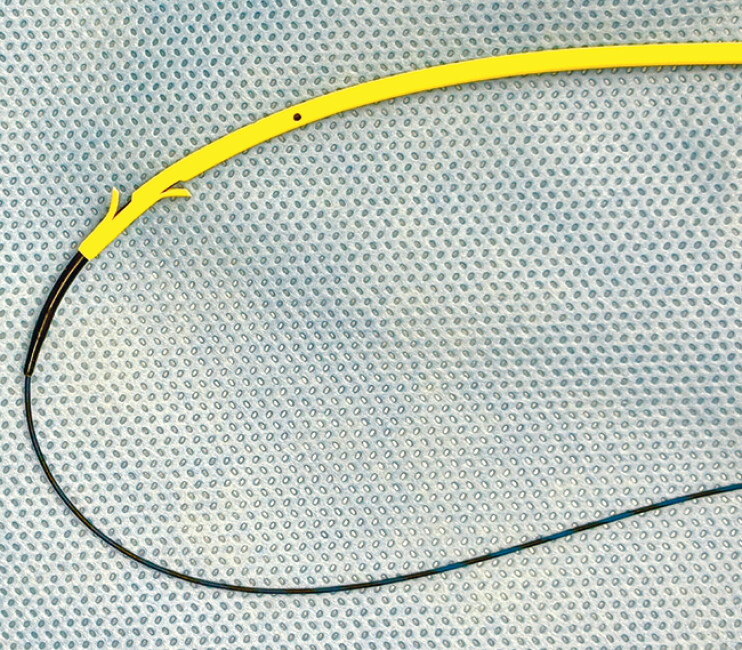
A novel 0.035-inch guidewire (CAPELLA 0.035) has a stiff shaft that facilitates stent deployment in endoscopic ultrasound-guided hepaticogastrostomy, and it is also available with most devices dedicated to 0.025-inch guidewires.
